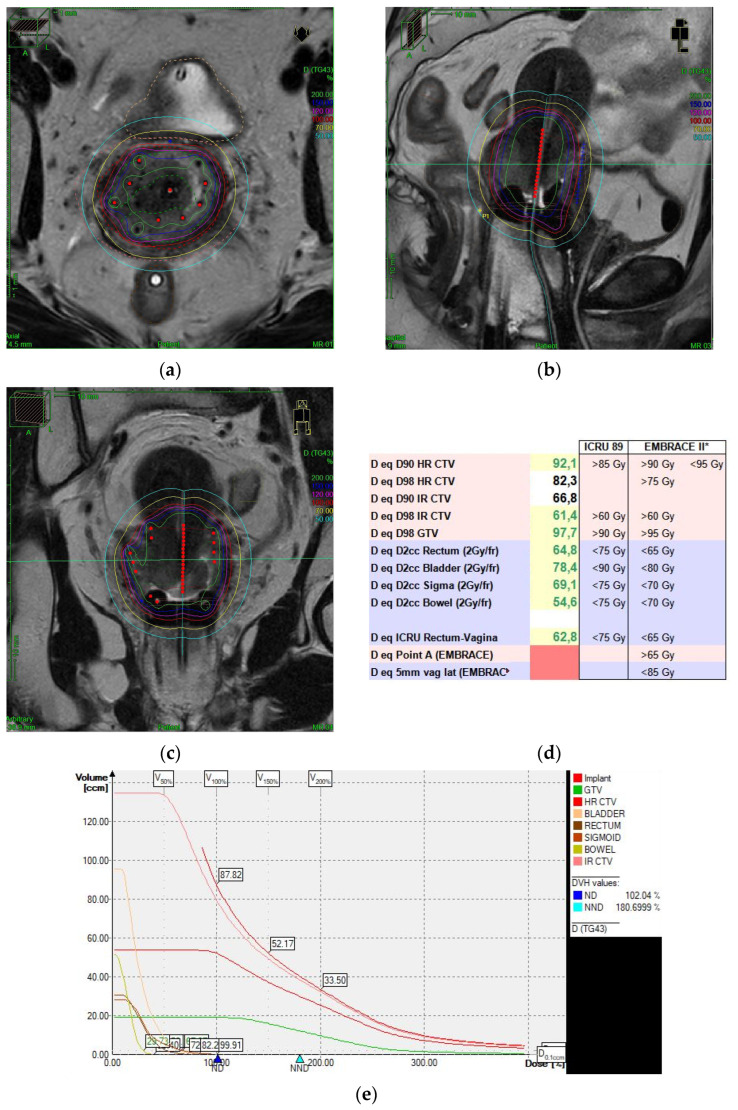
Image-guided adaptive brachytherapy (IGABT) for a patient with HPV-related squamous cell carcinoma of the cervix, T2bN2M0 FIGO 2018 stage IIIC2. Concomitant radiochemotherapy was delivered with 45 Gy in 25 fractions of 1.8 Gy, followed by high dose rate (HDR) MRI-guided IGABT in 4 fractions of 7 Gy/fraction, administered in two separate implants. A combined intracavitary/interstitial (IC/IS) technique was performed, using 10 round interstitial needles (8 parallel and 2 oblique). Panels (a–c) show axial, sagittal, and coronal views of the implant dosimetry using Oncentra^®^ Brachy version 4.6.3 (Elekta AB, Stockholm, Sweden), respectively. The images show full coverage of the large residual tumor and cervix with IC/IS implant, while sparing organs at risk (bladder, rectum, and sigmoid). Panel (d) displays the cumulative EQD2 doses, with D90 to the high-risk clinical target volume (HR-CTV) reaching 92.1 Gy. Panel (e) shows the dose–volume histogram (DVH) for the brachytherapy plan. The image was edited by the author and is reproduced with permission from the Radiation Oncology Department at ICO Hospitalet de Llobregat. * Planning aim and constraint recommendations based on the EMBRACE II study.
